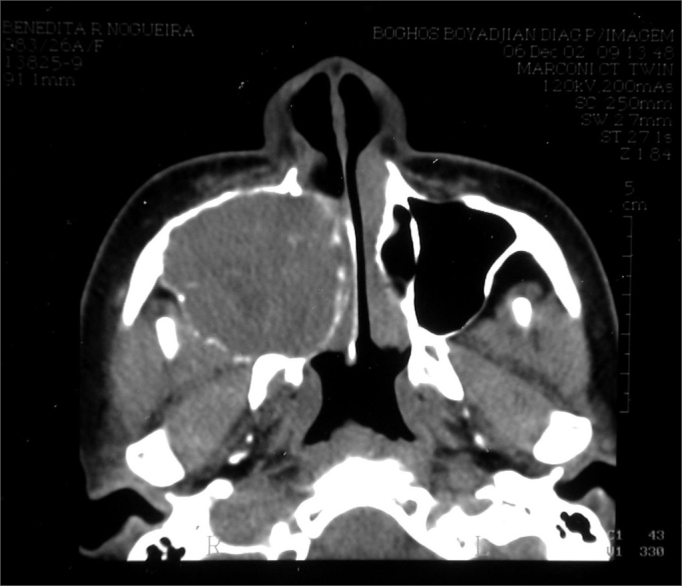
CT scan - axial cross section of a GCCG (case 2).
